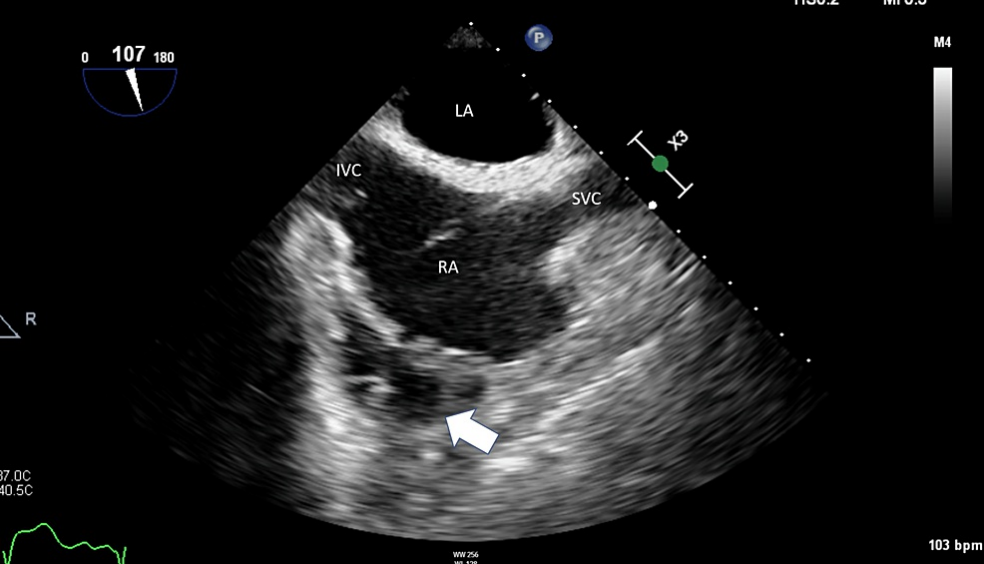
Two-dimensional TEE still frame showing the mid esophageal bicaval view (107°) during mid systole, demonstrating a loculated pericardial effusion (arrow) adjacent to the RA TEE: Transesophageal echo; IVC: Inferior vena cava; LA: Left atrium; RA: Right atrium; SVC: Superior vena cava
